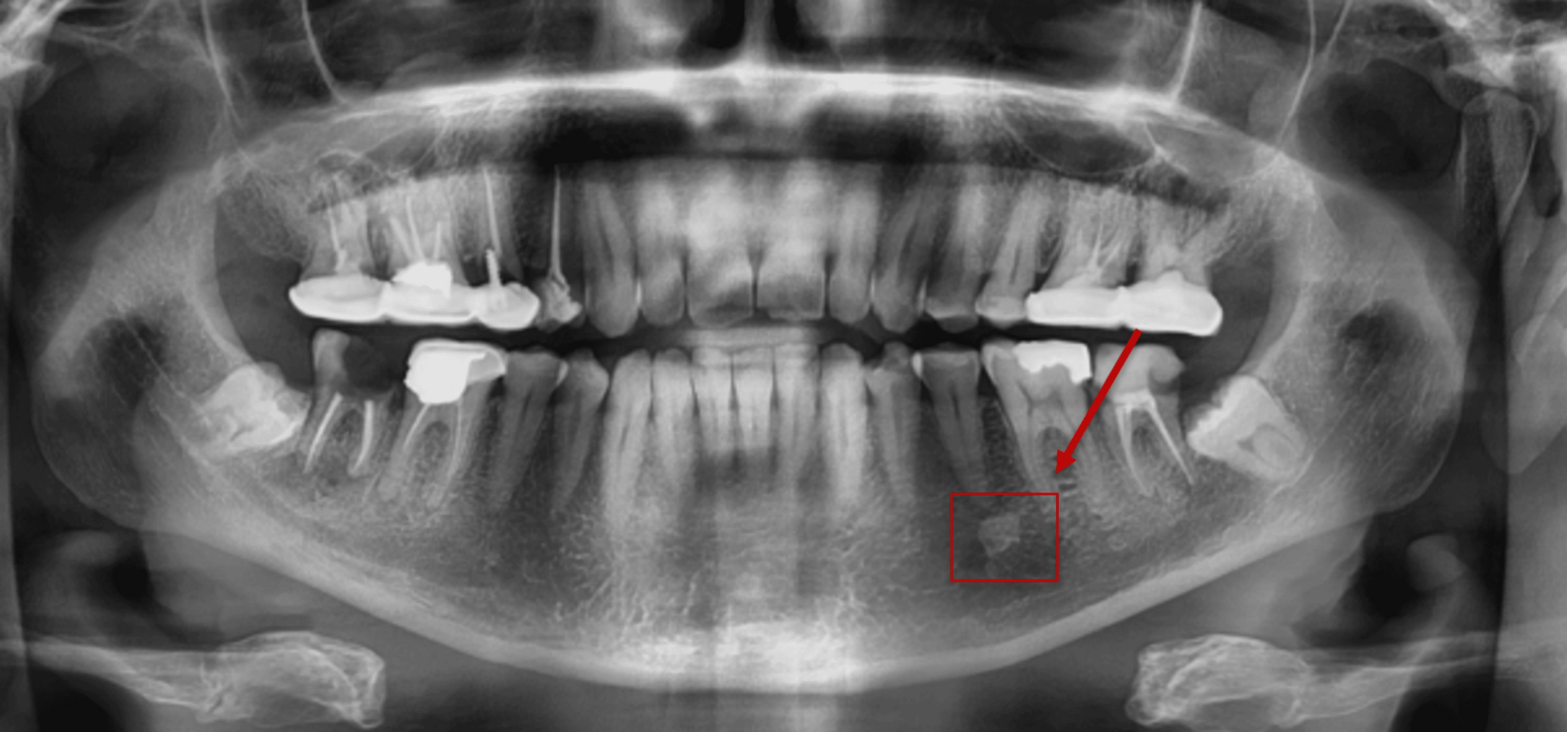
A panoramic radiograph of a 40-year-old male patient showing a periapical radiopaque lesion on the left side of the mandibular posterior region.
